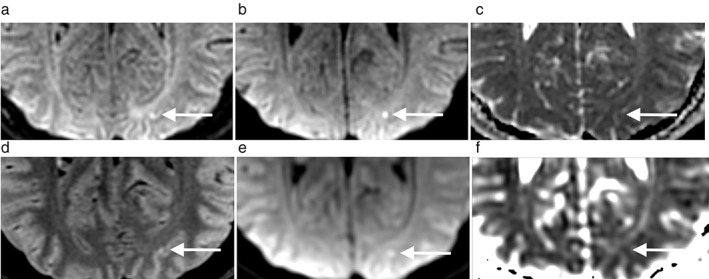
False‐negative finding on routine MRI. A 35‐year‐old man with left vertebral dissection presenting with motor and sensory deficits in left hand and arm. On EPIMix (upper row) DWI (b) shows a 3 mm hyperintense DWI lesion (white arrow) in the left occipital lobe, with a slight hyperintensity on T2‐FLAIR (a) and not visible on the ADC map (c). The suspected lesion is barely visible on reconstructed images of routine clinical MRI T2‐FLAIR (d) and DWI (e) and not visible on ADC (f). The ischemic lesion was detected by both readers on EPIMix but missed on routine MRI.
